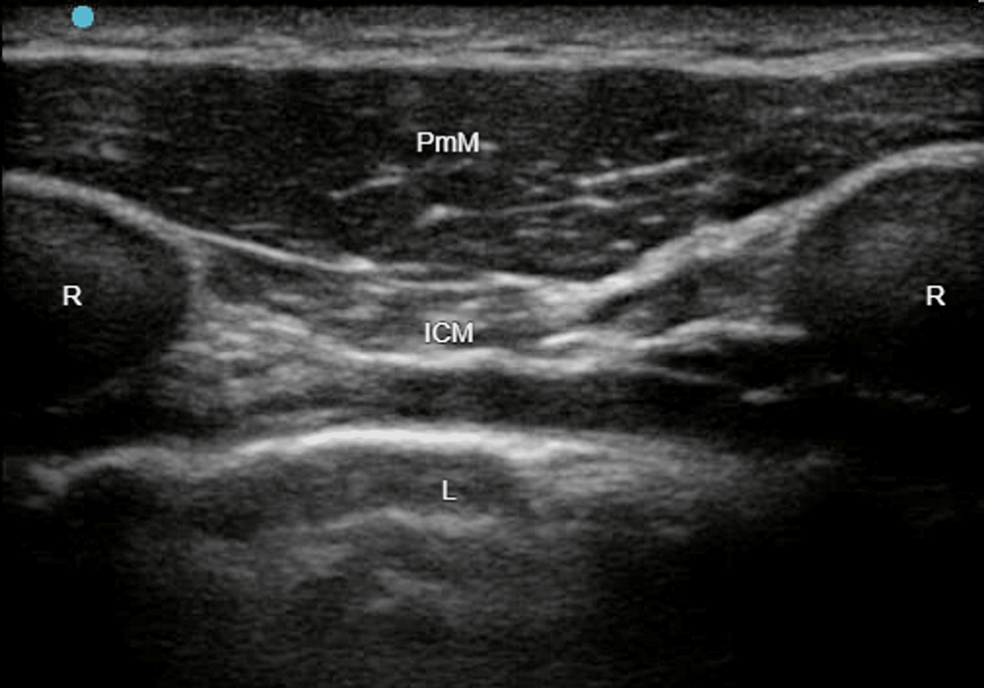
Parasternal block (PSB). PmM: pectoralis major muscle. ICM: intercostal muscles. R: rib. L: lung.
